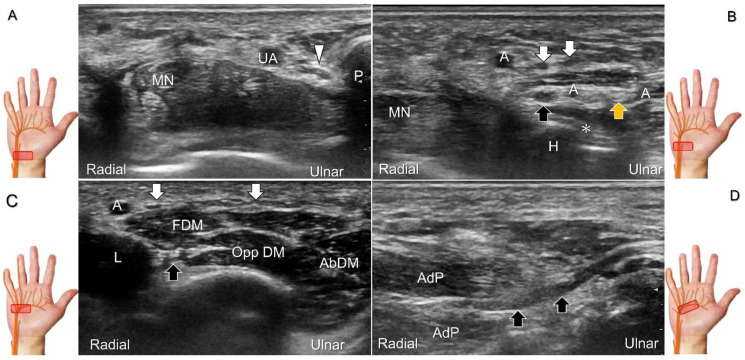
Sonographic imaging (short-axis view) shows the ulnar nerve within the Guyon’s canal (A), separation of branches beside the pisohamate hiatus (B), deep branch of the ulnar nerve located between the hypothenar muscles distal to the hook of the hamate (C). Long-axis view (through pivoting the transducer) shows the segment of the deep branch within the adductor pollicis muscle (D). Arrowhead: ulnar nerve; white arrows: superficial branch of the ulnar nerve; black arrows: deep branch of the ulnar nerve; orange arrow: branch of the ulnar nerve to the abductor digiti minimi; *: pisohamate ligment MN: median nerve; UA: ulnar artery; P: pisiform; H: hook of hamate; A: artery; L: lumbrical muscle; FDM: flexor digiti minimi brevis muscle; Opp DM: opponens digiti minimi muscle; AbDM: abductor digiti minimi muscle; AdP: adductor pollicis muscle.
